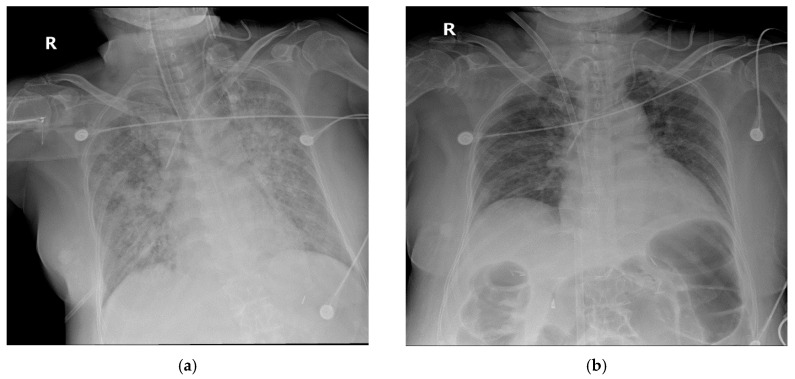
Chest radiography of the patient 2. (a) on the day of ECMO application (b) on the day of ECMO discontinuation.
